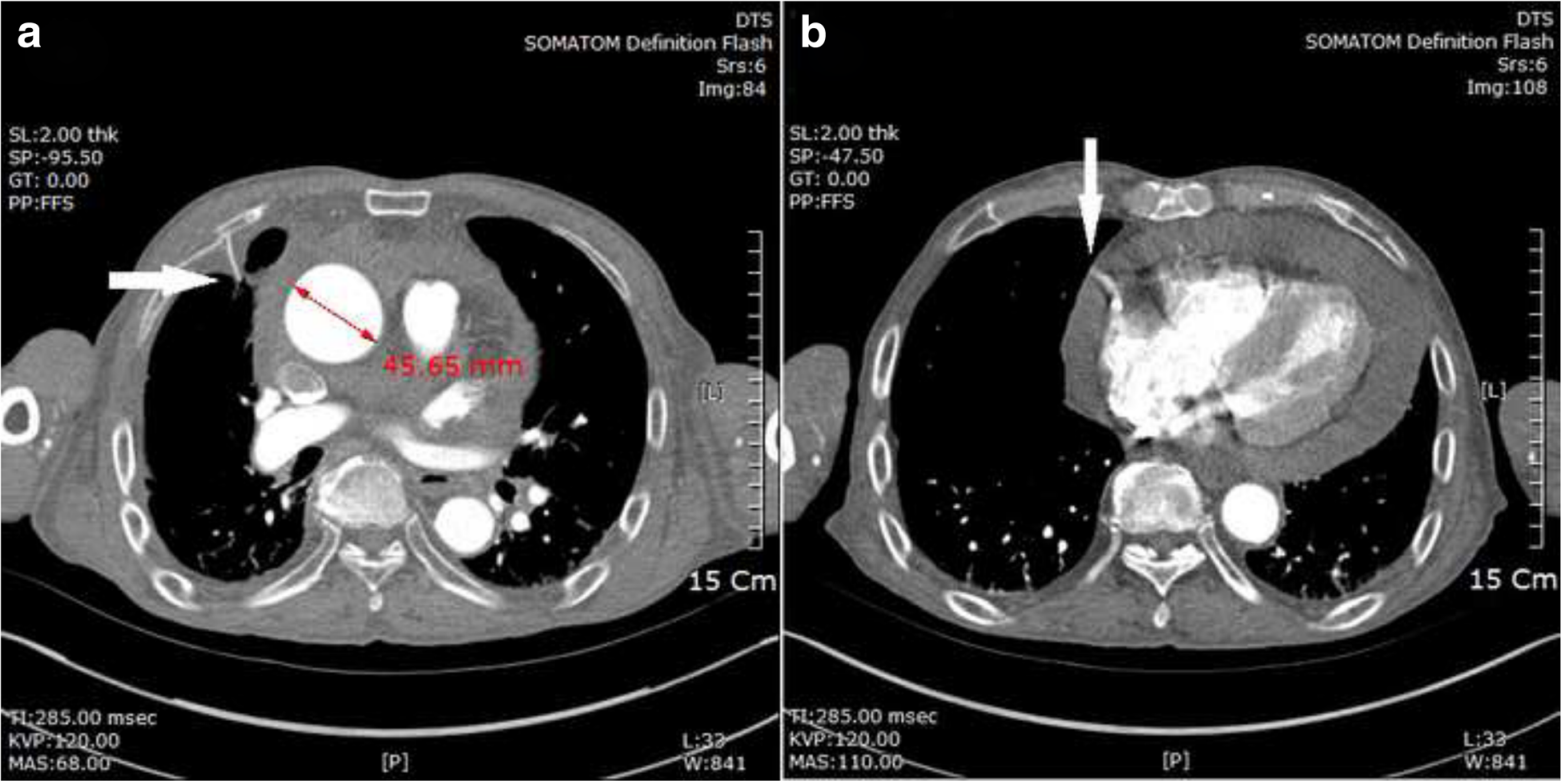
a Bony fragment of the fractured 4th rib (*arrow*). The ascending aorta was measured to be about 45 mm in maximum diameter. b Contrast-enhanced computed tomography showing collection of hemorrhagic fluid in the pericardium, with enhancement in the *right side* of the heart, suggesting leakage of contrast medium (*arrow*)
